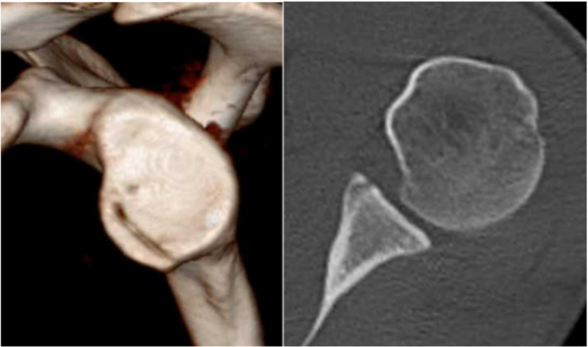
No loss of glenoid surface area or diameter can be detected on the preoperative 3DCT scans. However, a dimished glenoid concavity can be detected on the axial CT scans
